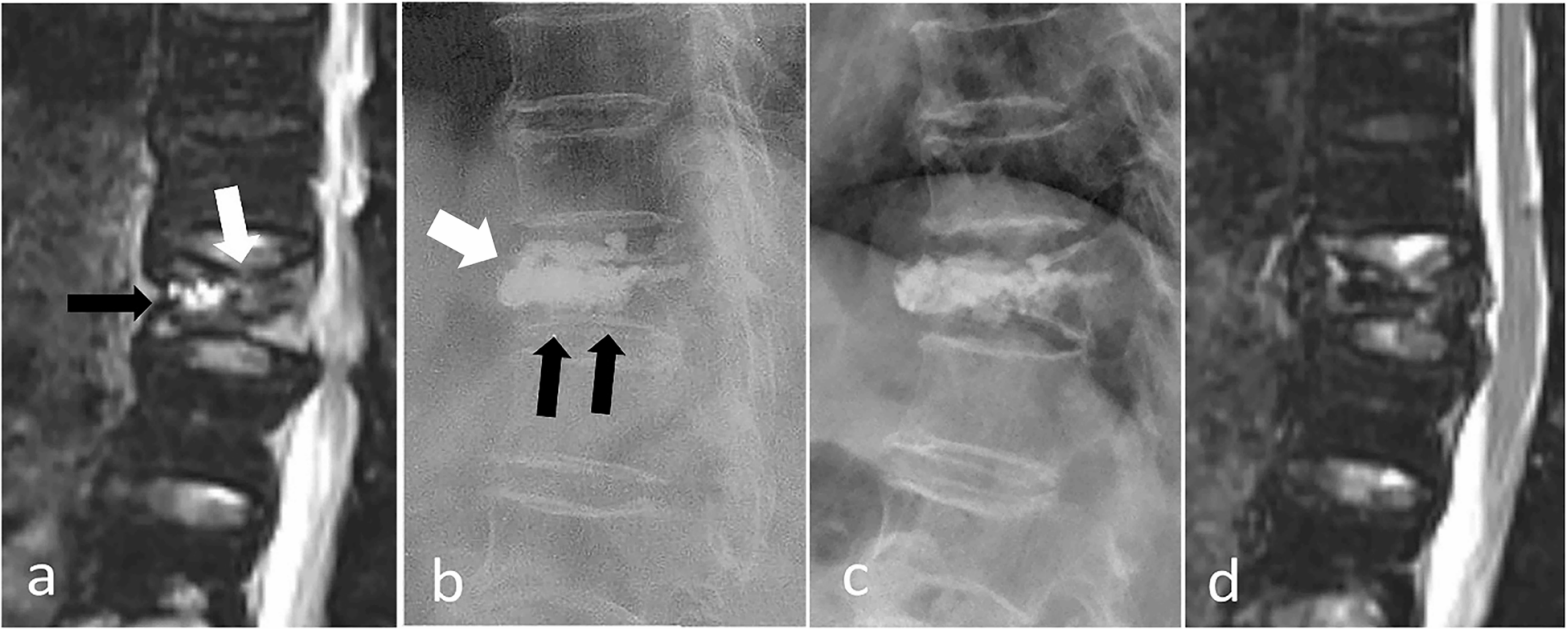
An 82-year-old woman with refracture after PVP. MRI and lateral plain X-ray of an 82-year-old woman with a T11 compression fracture. a Sagittal SPAIR showed a T11 compression fracture with bone marrow edema, IVC (black arrow), and endplate cortical disruption (white arrow). b Postoperative X-ray showed the cemented vertebrae with NPEC on the lower endplate (black arrow) and displacement of the anterior edge of the vertebral body (white arrow). c-d MRI and lateral X-ray at 5 months after PVP showed loss of height of T11 and bone marrow edema
